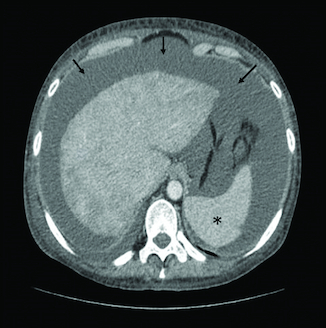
Liver dysmorphia and signs of portal hypertension (splenomegaly [asterisk] and ascites [arrow]) on abdominal CT scan.
